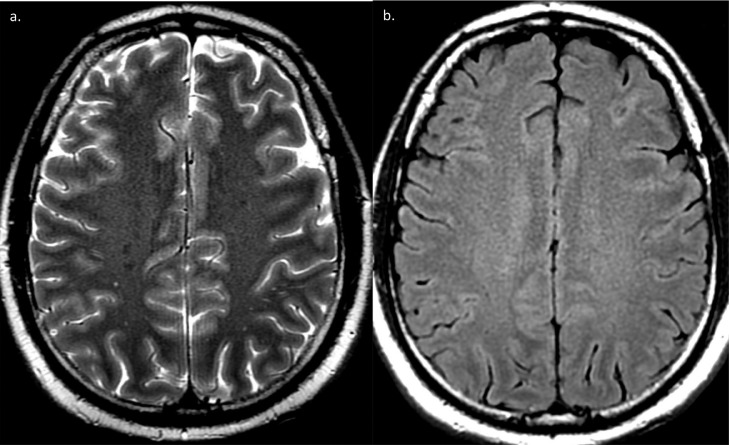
T2 (a) and Flair (b) MRI sequences at follow up MRI, six days after initial MRI showing no detectable signal changes.
